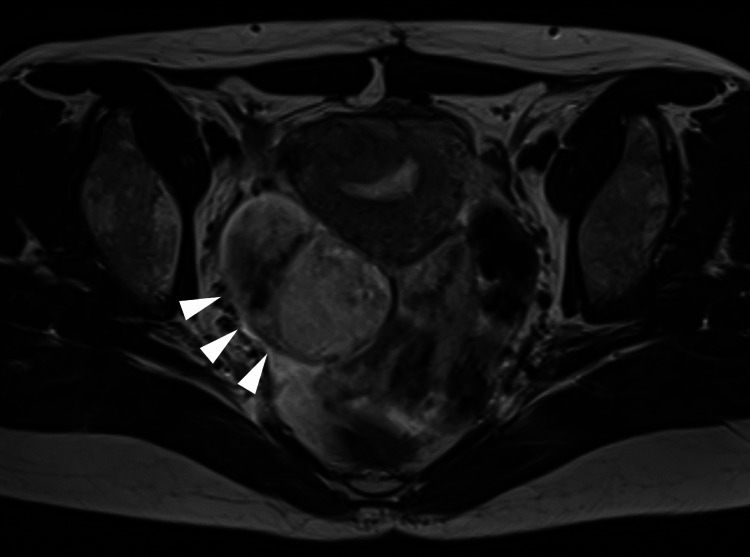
T2-weighted magnetic resonance imaging scan (transverse section) showing right ovarian tumor, suggesting the recurrence of serous borderline tumor (arrowheads).
